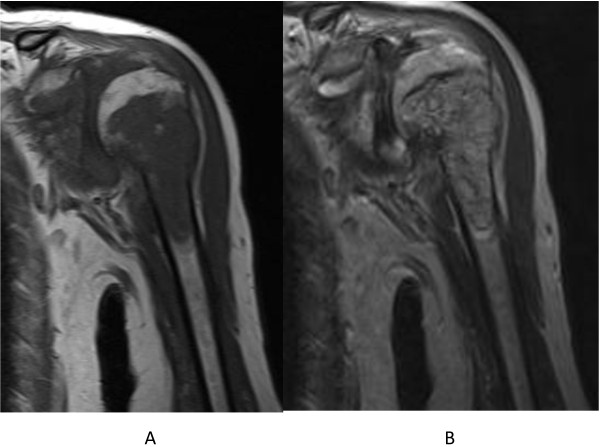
Magnetic resonance imaging (MRI) of the left proximal humerus. (A) Sagittal T1-weighted MRI shows a low-signal-intensity tumor replacing bone marrow. (B) Coronal T2-weighted MRI demonstrates an increase in the signal intensity of the tumor.
